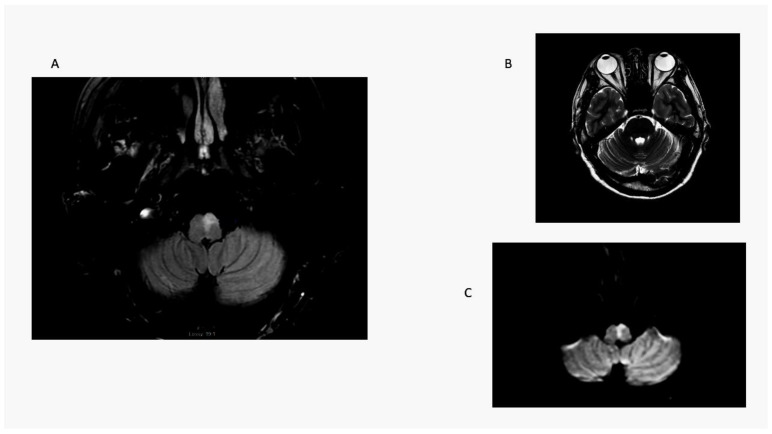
Medial medullary stroke. Panel (A): axial T2 MRI shows an elongated paramedian area of hyperintensity involving the left medullary pyramid and extending toward the dorsal medulla near the floor of the fourth ventricle. Note absence of radiographic ocular deviation in a T2 axial MRI (Panel (B)). Panel (C) is a DWI scan showing restricted diffusion confirmed by ADC map. Here, a combination of right-sided weakness and grade 3 ataxia causes contralesional falls.
